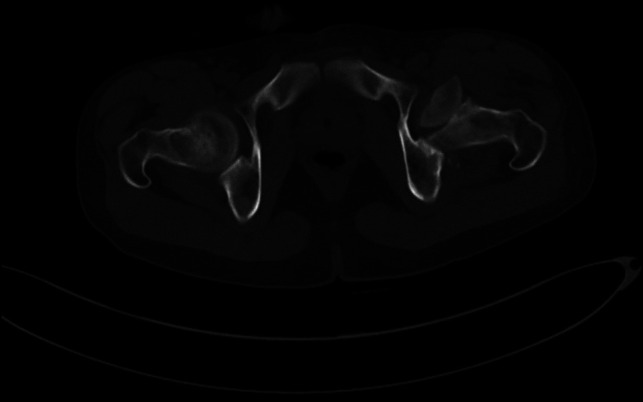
Postreduction preoperative CT scan showing the fracture and the rotated left femoral head fragment.
